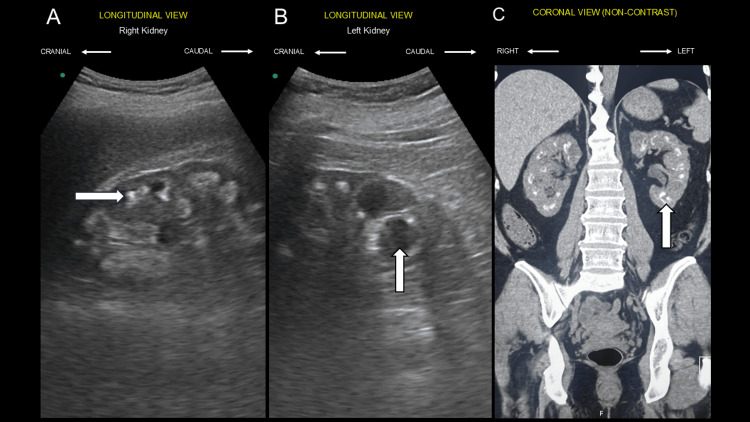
Renal imaging findings on point-of-care ultrasound and computed tomography (A) Point-of-care ultrasound of the right kidney demonstrating multiple medullary cysts, echogenic pyramids, and hyperechoic foci with posterior acoustic shadowing (arrow indicates a medullary cyst containing hyperechoic foci consistent with calculi). (B) Point-of-care ultrasound of the left kidney showing larger medullary cysts and calcifications (arrow indicates a medullary cyst containing hyperechoic foci consistent with calculi). (C) Non-contrast computed tomography of the abdomen demonstrating bilateral curvilinear calcifications of the medullary pyramids and medullary cysts consistent with nephrocalcinosis (arrow indicates medullary calcification/calculus).
